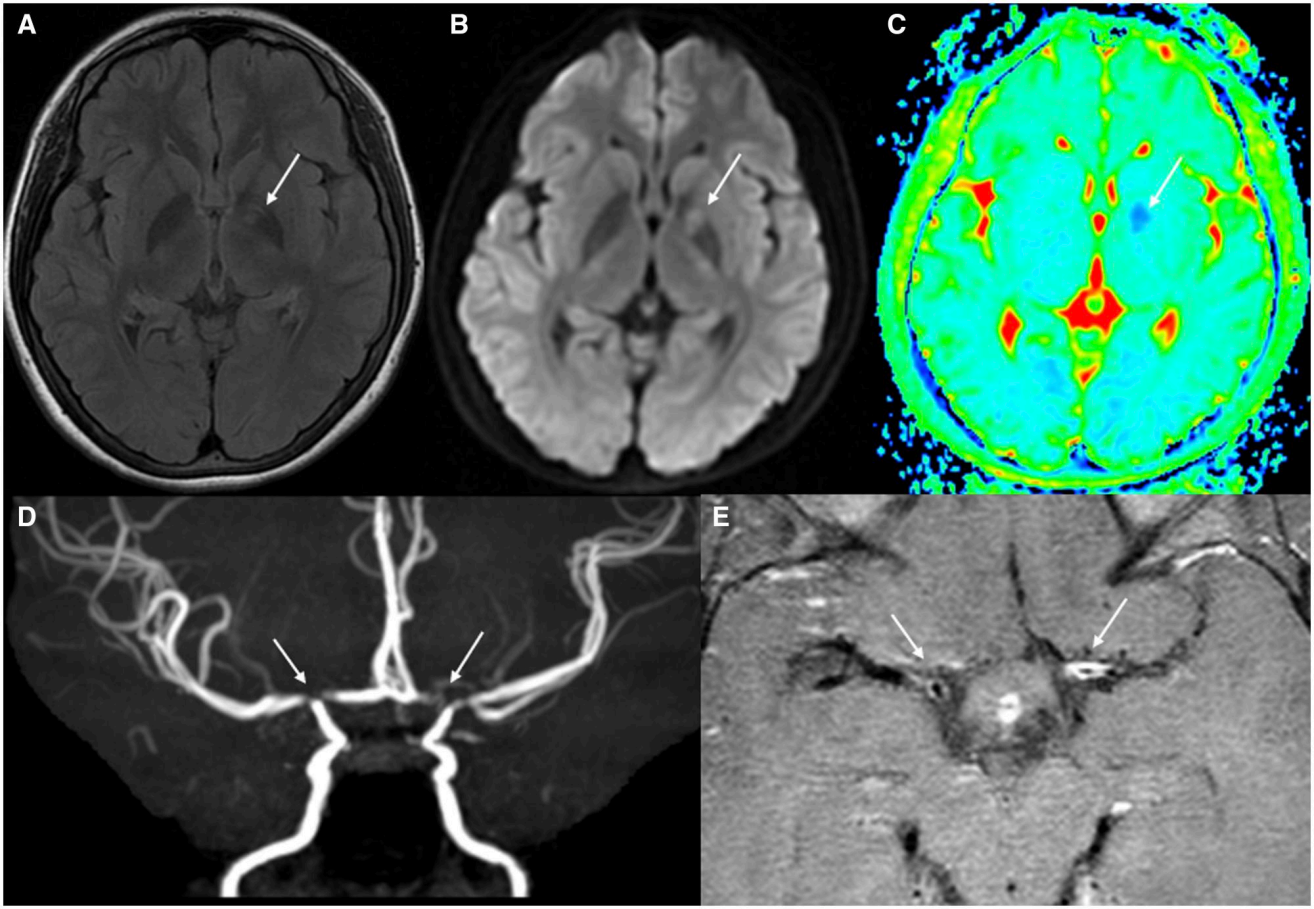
A 15-year-old female SLE patient. Right limb weakness with slurred speech for 15 days, ANA and aPL antibody positive. MRI: (A) T2-weighted FLAIR, (B) DWI, (C) ADC; acute infarction in the left basal ganglia. (D) MRA: severe stenosis of the lumen of the bilateral ICA C7 segments, bilateral ACA A1 segments and bilateral MCA M1 segments. (E) HR-VWI: bilateral ICA C7 segments, bilateral ACA A1 segments, bilateral MCA M1 segments with wall thickening, high enhancement and stenosis grade 3
